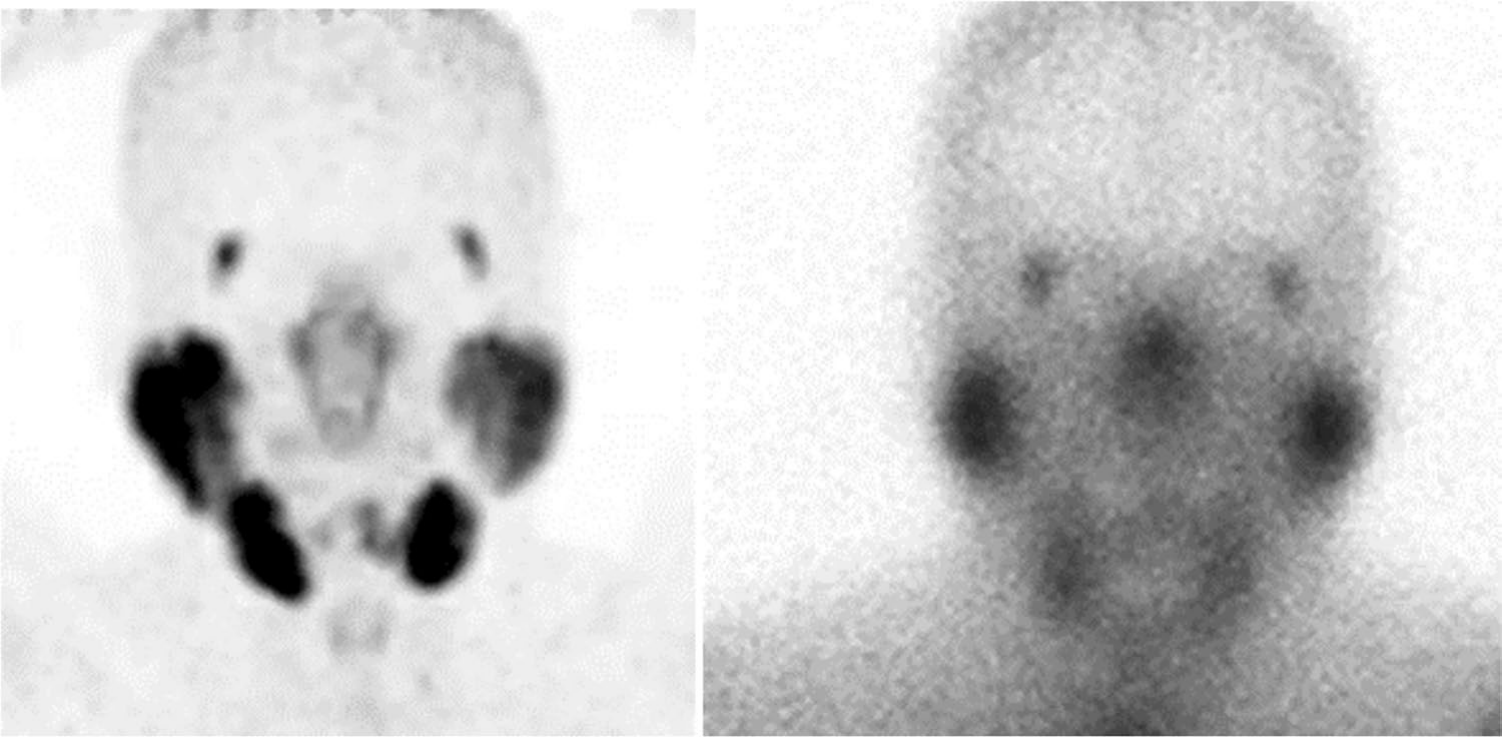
Anterior maximum-intensity projection of pre-therapy [68Ga]Ga-PSMA PET/CT (left) and [177Lu]Lu-PSMA-therapy gamma camera image (right) in a patient treated for metastatic prostate cancer. Large uptake can be observed in the different salivary glands and in lacrimal glands
